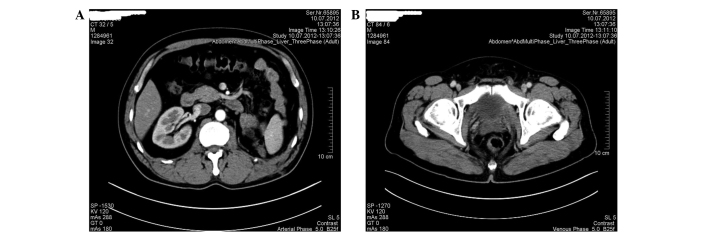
Abdominal computed tomography (CT) scan following the second surgery showed no evidence of recurrence or metastasis. (A) abdominal CT and (B) pelvic CT.
